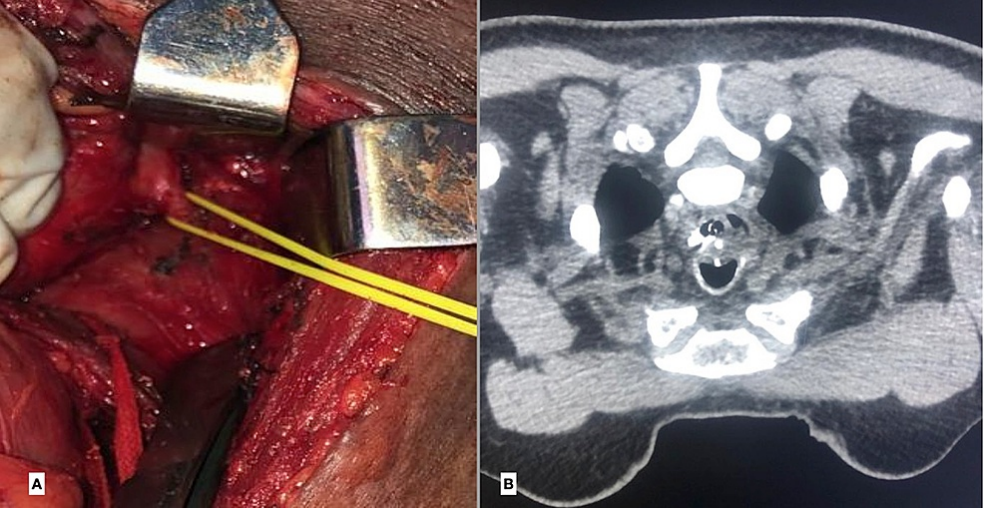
(A) Intra-operative photo showing the fistula looped with a vascular sling, (B) prone CT image post-repair showing the staples in trachea and the esophagus with buttressed sternocleidomastoid muscle in between the two with no evidence of contrast leak.
